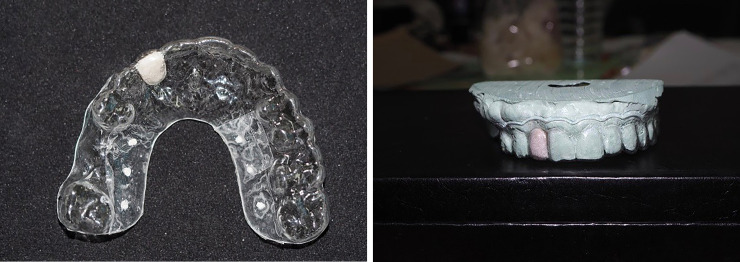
Vacuum radiographic template. (A) Barium sulfate was used as a radiopaque marker around the future prosthesis. (B).
